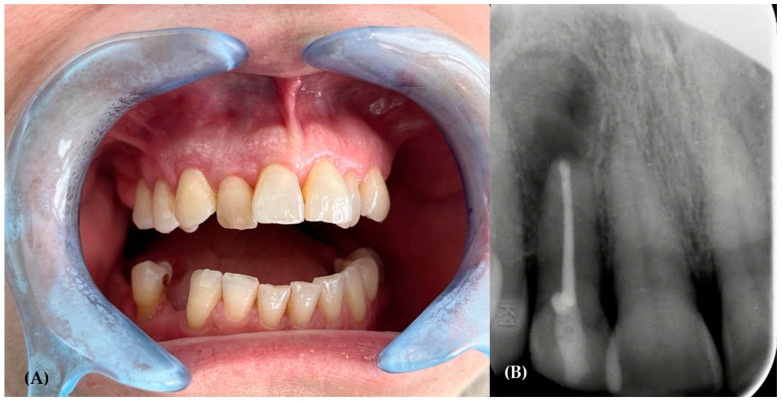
(A) Intraoral image after 3 months of healing; tissues appeared normal. (B) Endoral radiograph showing the process of bone healing.
